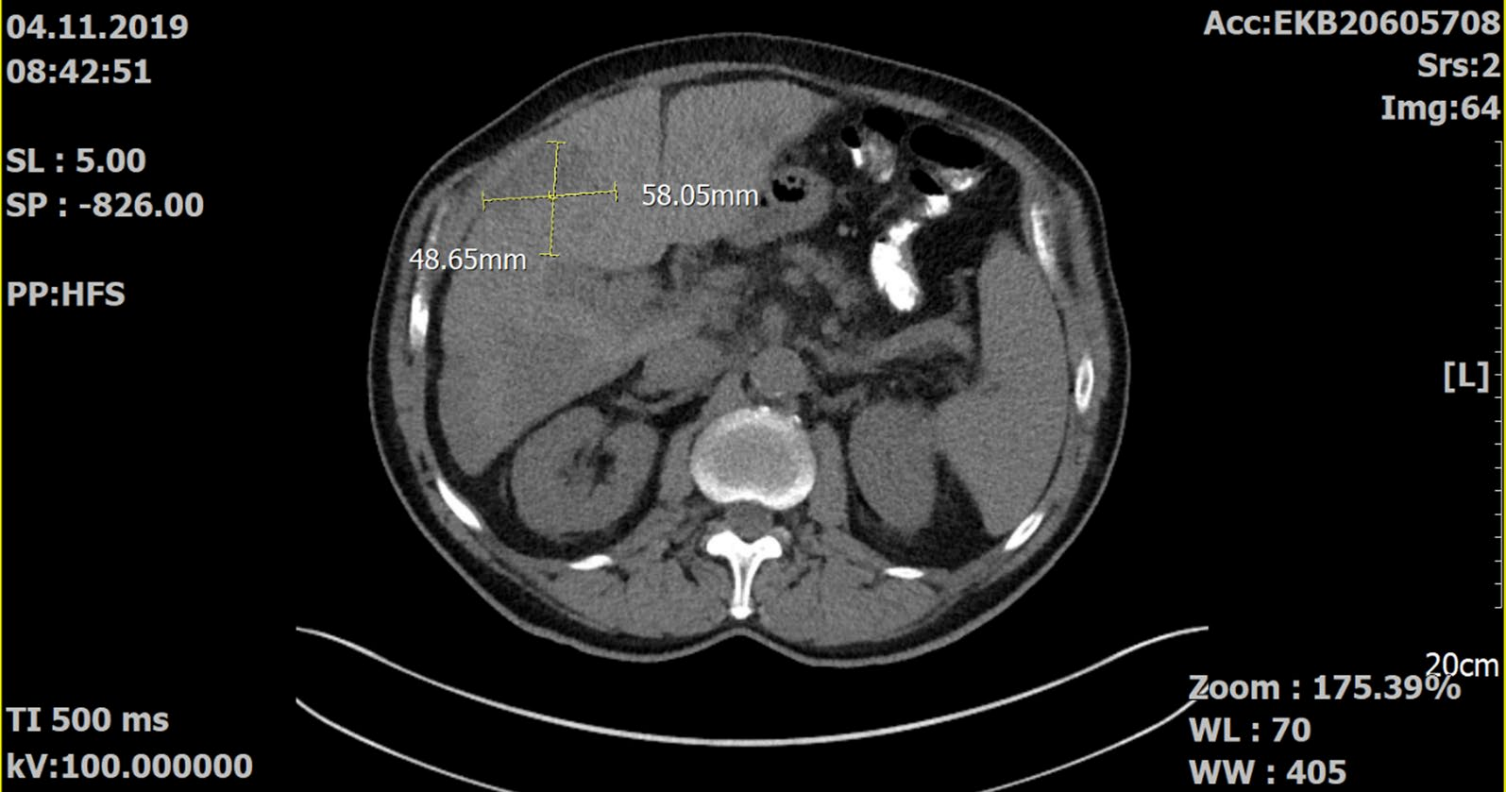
Selected CT images of the abdomen performed on November 2019 and August 2021
